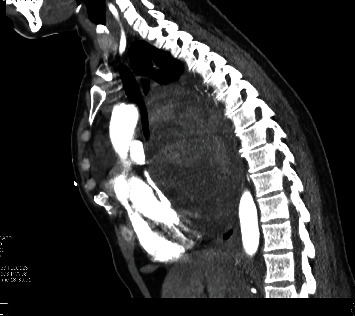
CT sagittal projection centered along the midline depicted anterior displacement of mediastinal structures secondary to larger left hemithoracic mass, which crosses the midline.
